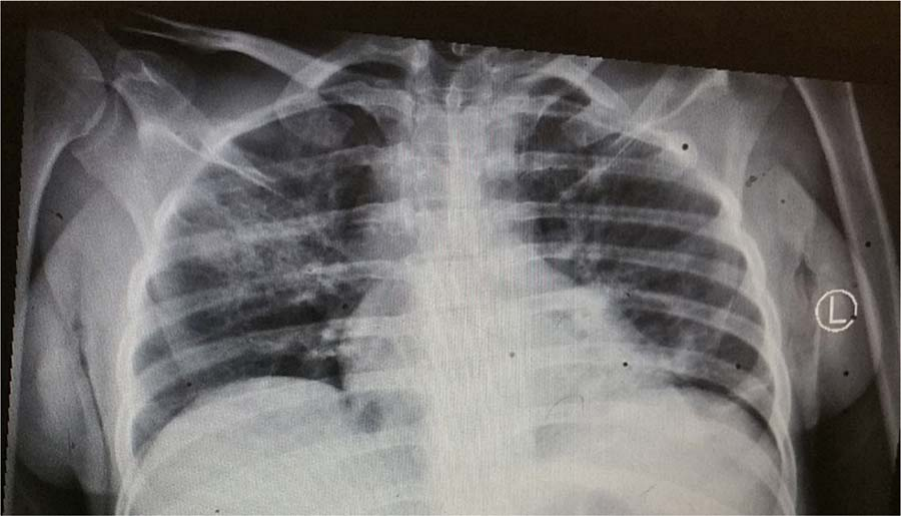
Chest X-ray showing consolidations in the right lung.
